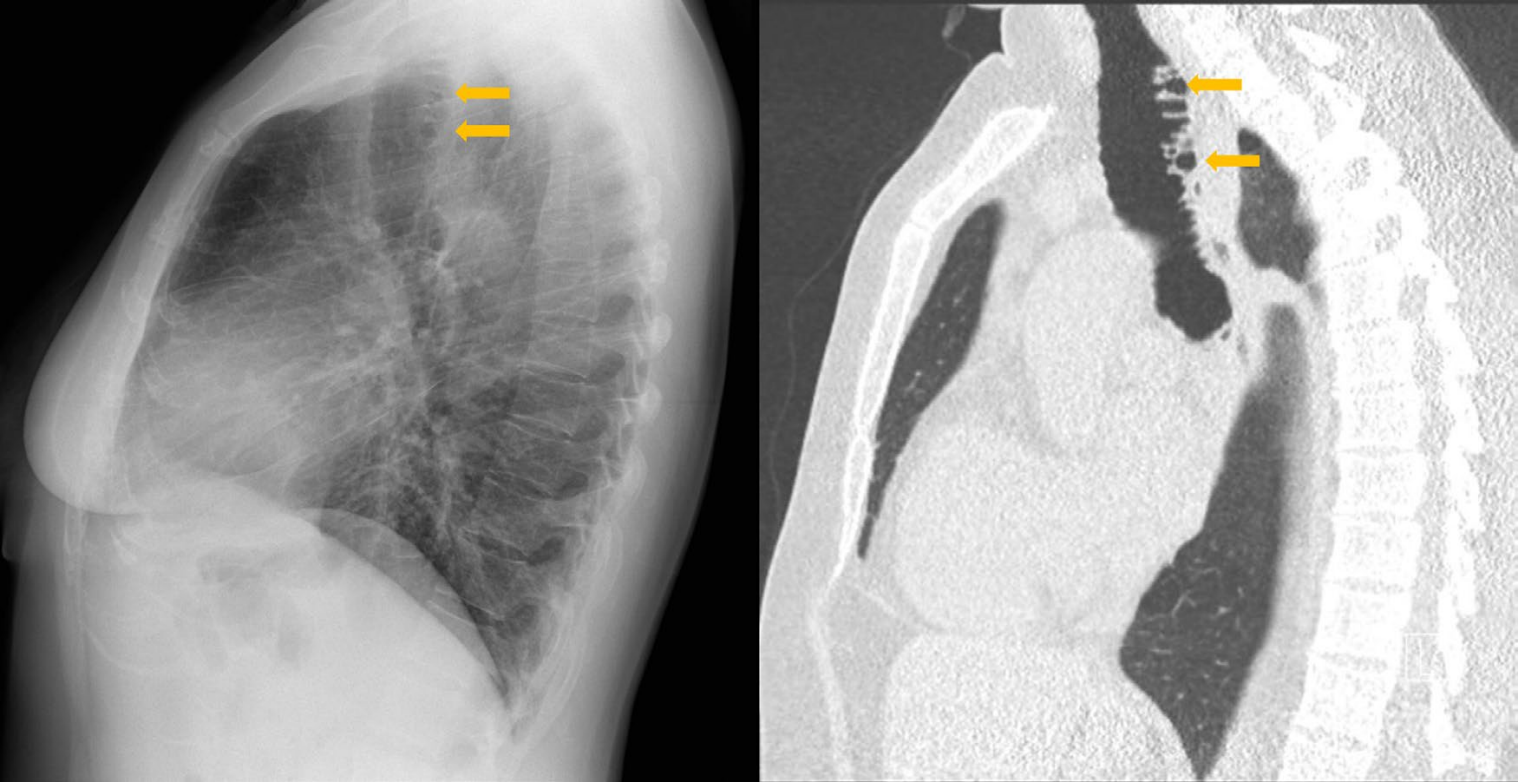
Tracheal diverticulosis: sagital CXR and CT comparison.
